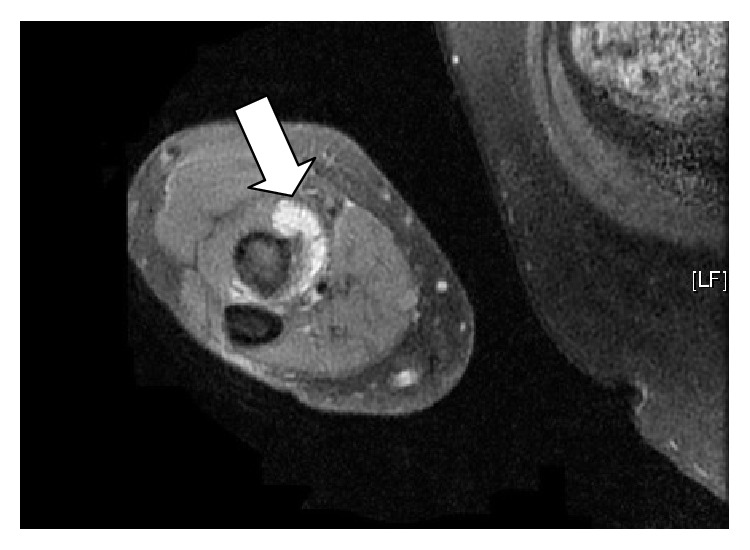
MRI axial T2 weighted image after IV gadolinium clearly highlighting the bursa structure (thick arrow).
